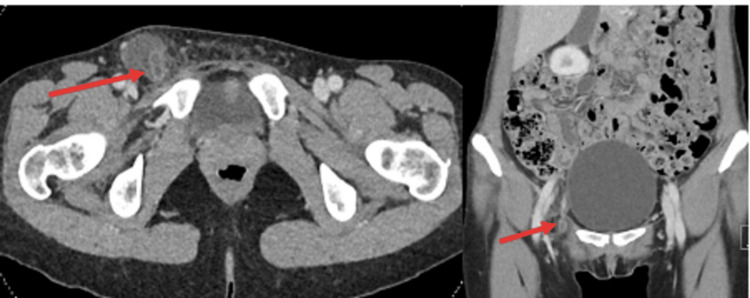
Preoperative computed tomography showed a right femoral hernia containing acute appendicitis
